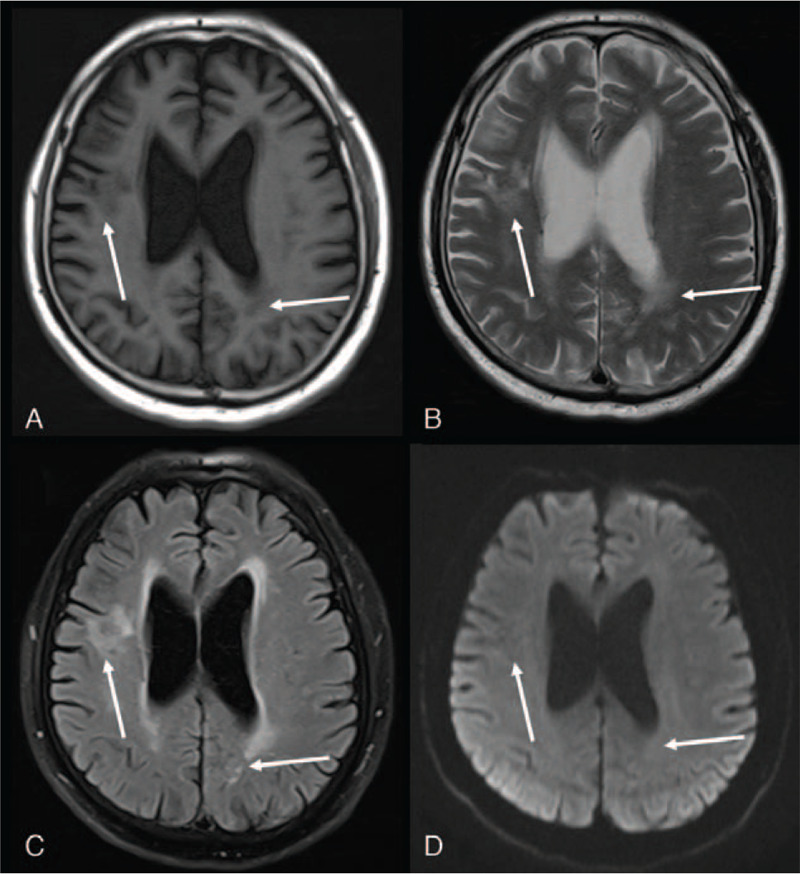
Reexamination of the brain MRI one and a half years later showed resolution of lesions with small softened foci and glial hyperplasia. A, Axial T1-weighted image. B, Axial T2-weighted image. C, Axial T2 Flair-weighted image. D, Diffusion-weighted image (b = 800). MRI = magnetic resonance imaging.
